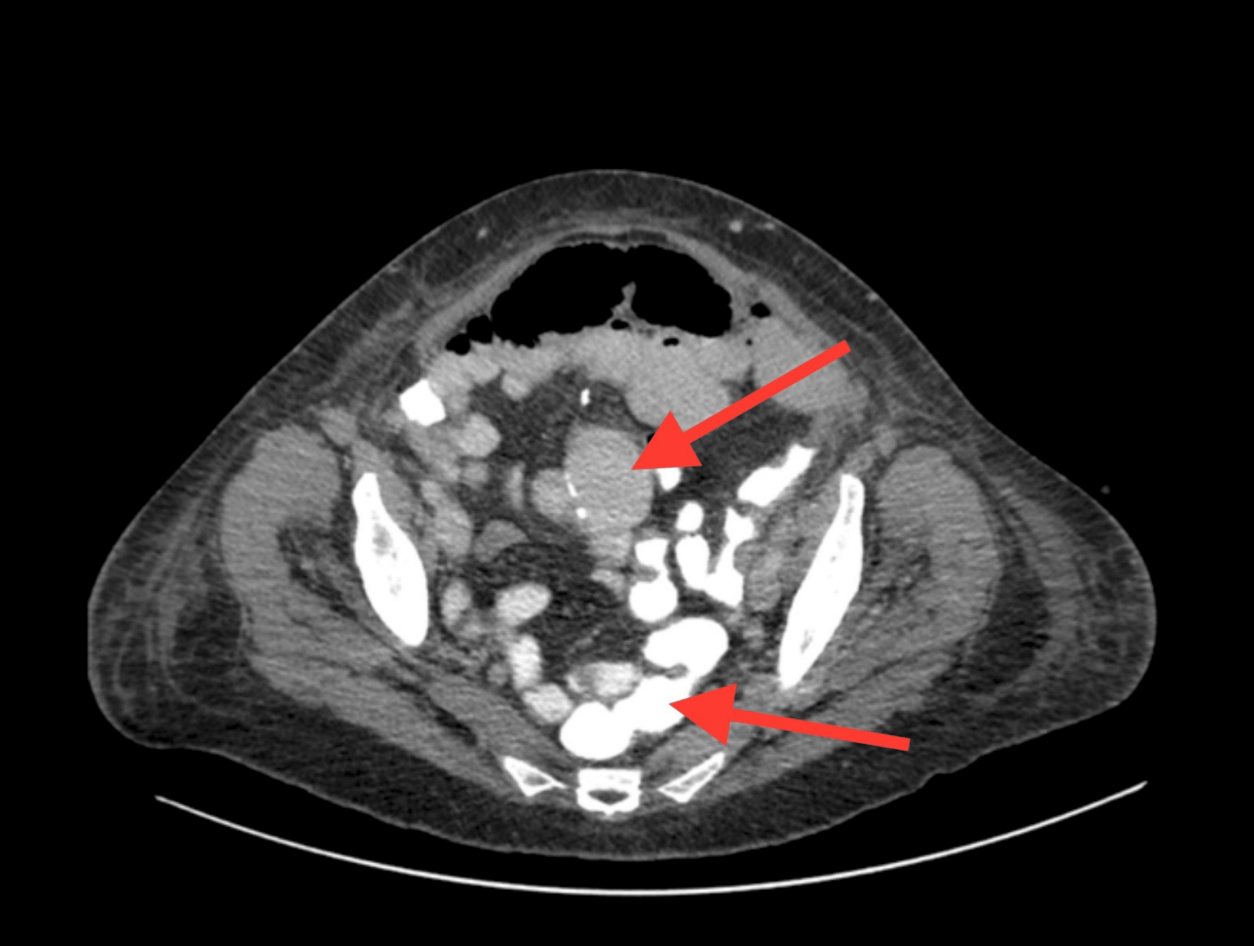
Abdominal CT with gastrografin day 6: Findings indicated persistent proximal dilatation, some gastrografin passed distally in the collapsed bowel, no signs of leak or collection Abdominal CT with oral contrast (gastrografin) on postoperative day 6 demonstrating persistent proximal small bowel dilatation. A small amount of contrast (bright fluid) has passed distally beyond the anastomosis into the collapsed bowel, and there are no signs of an anastomotic leak or intra-abdominal collection
